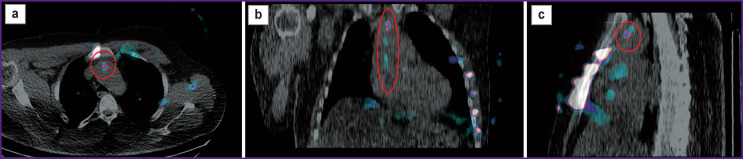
The resulting SPECT/CT images of the chest of patient P. (axial (a), frontal (b), and sagittal (c) slices), obtained after performing subtraction of the baseline images shown in Figure 2 The resulting SPECT/CT images show no significant artifacts from the blood pooled in the large vessels and the heart cavities, as well as from the sternum, which made it possible to visualize pathological focal RP accumulation in the ascending aorta wall (circled in red)
